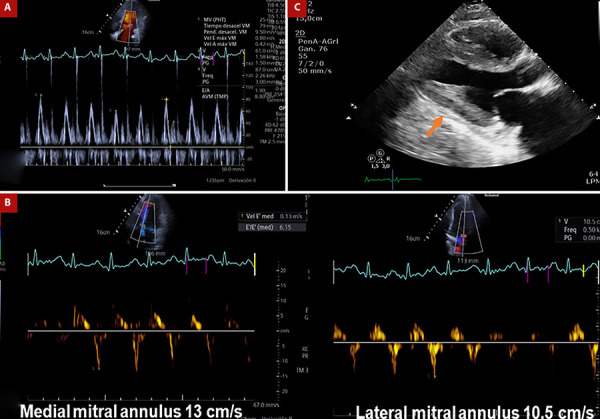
(A) Pulsed-wave Doppler spectrum of mitral inflow velocities demonstrates a marked respiratory variation of the peak E-wave velocity with short deceleration time. (B) Medial and lateral mitral annulus early diastolic (e’) velocities by tissue Doppler show a preserved longitudinal function and reversal of the usual relationship (so-called annulus reversus). (C) Thickened pericardium (arrow).
